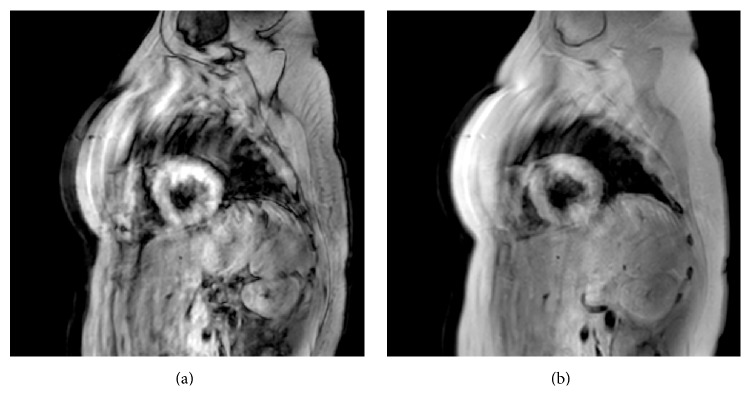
Cardiac magnetic resonance imaging: sequences HASTE (short axis) showing a hypersignal in the anterior wall (a) on the 5th day and (b) on the 60th day of aluminium phosphide poisoning.
